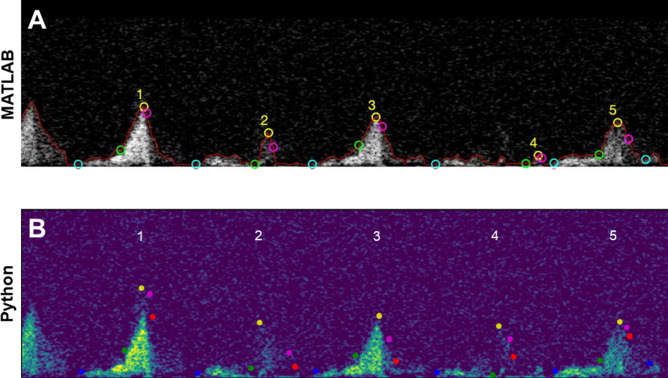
Figures displaying partially and completely captured fainter cycles in the Doppler region. Panel (A) above shows cycles 2 and 4 are not fully captured by the MATLAB program, but they are captured and analyzed by the Python program in Panel (B) below. As in previous images, green points indicate the beginning of the diastolic phase, yellow indicates peak velocity, pink indicates decay velocity, red indicates peak diastolic deceleration, and blue points indicate the end of the cycle.
